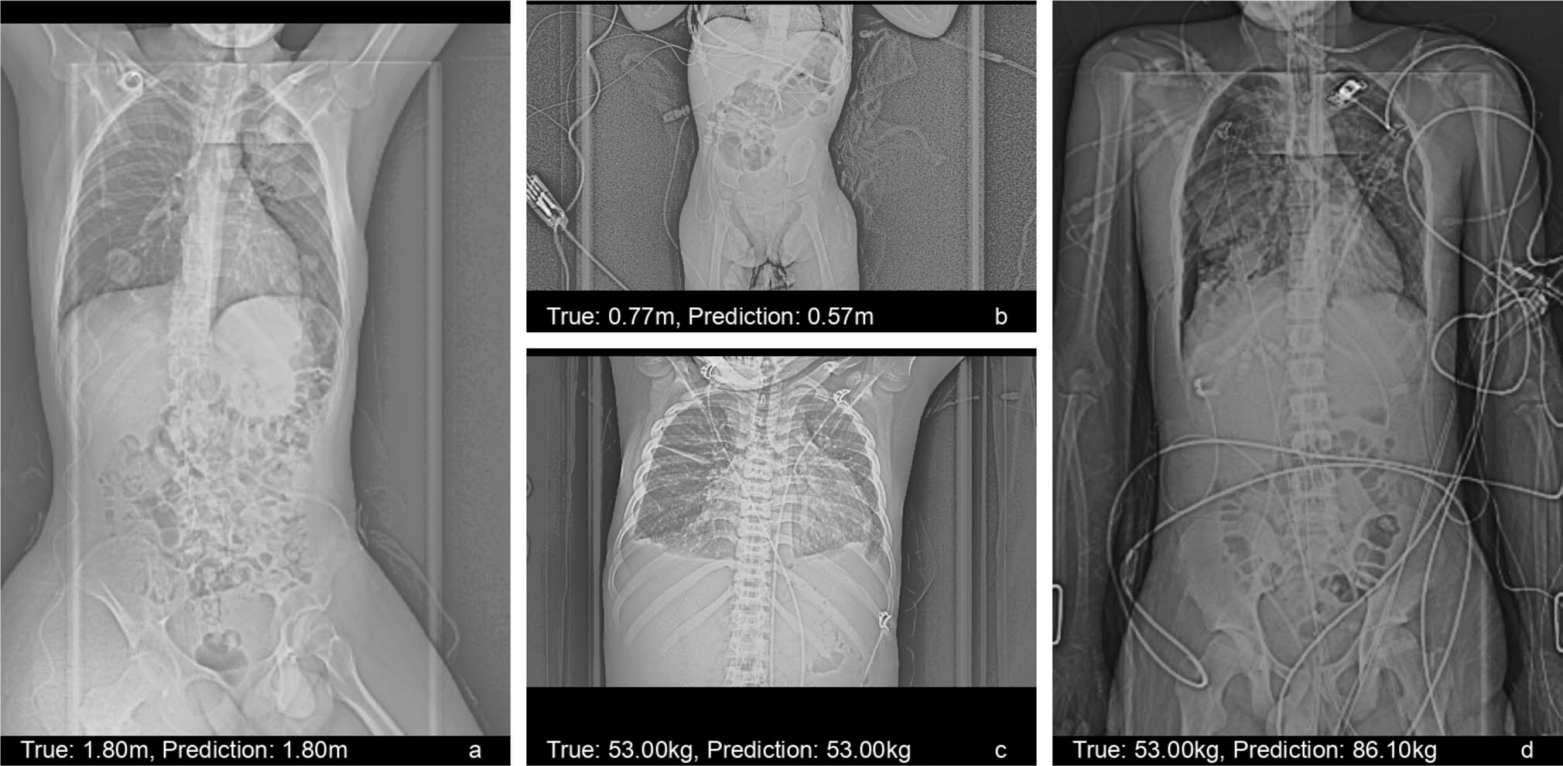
Example CT localizers with best and worst predictions. (a) Prediction with lowest height error. (b) Prediction with highest height error. (c) Prediction with lowest weight error. (d) Prediction with highest weight error.
